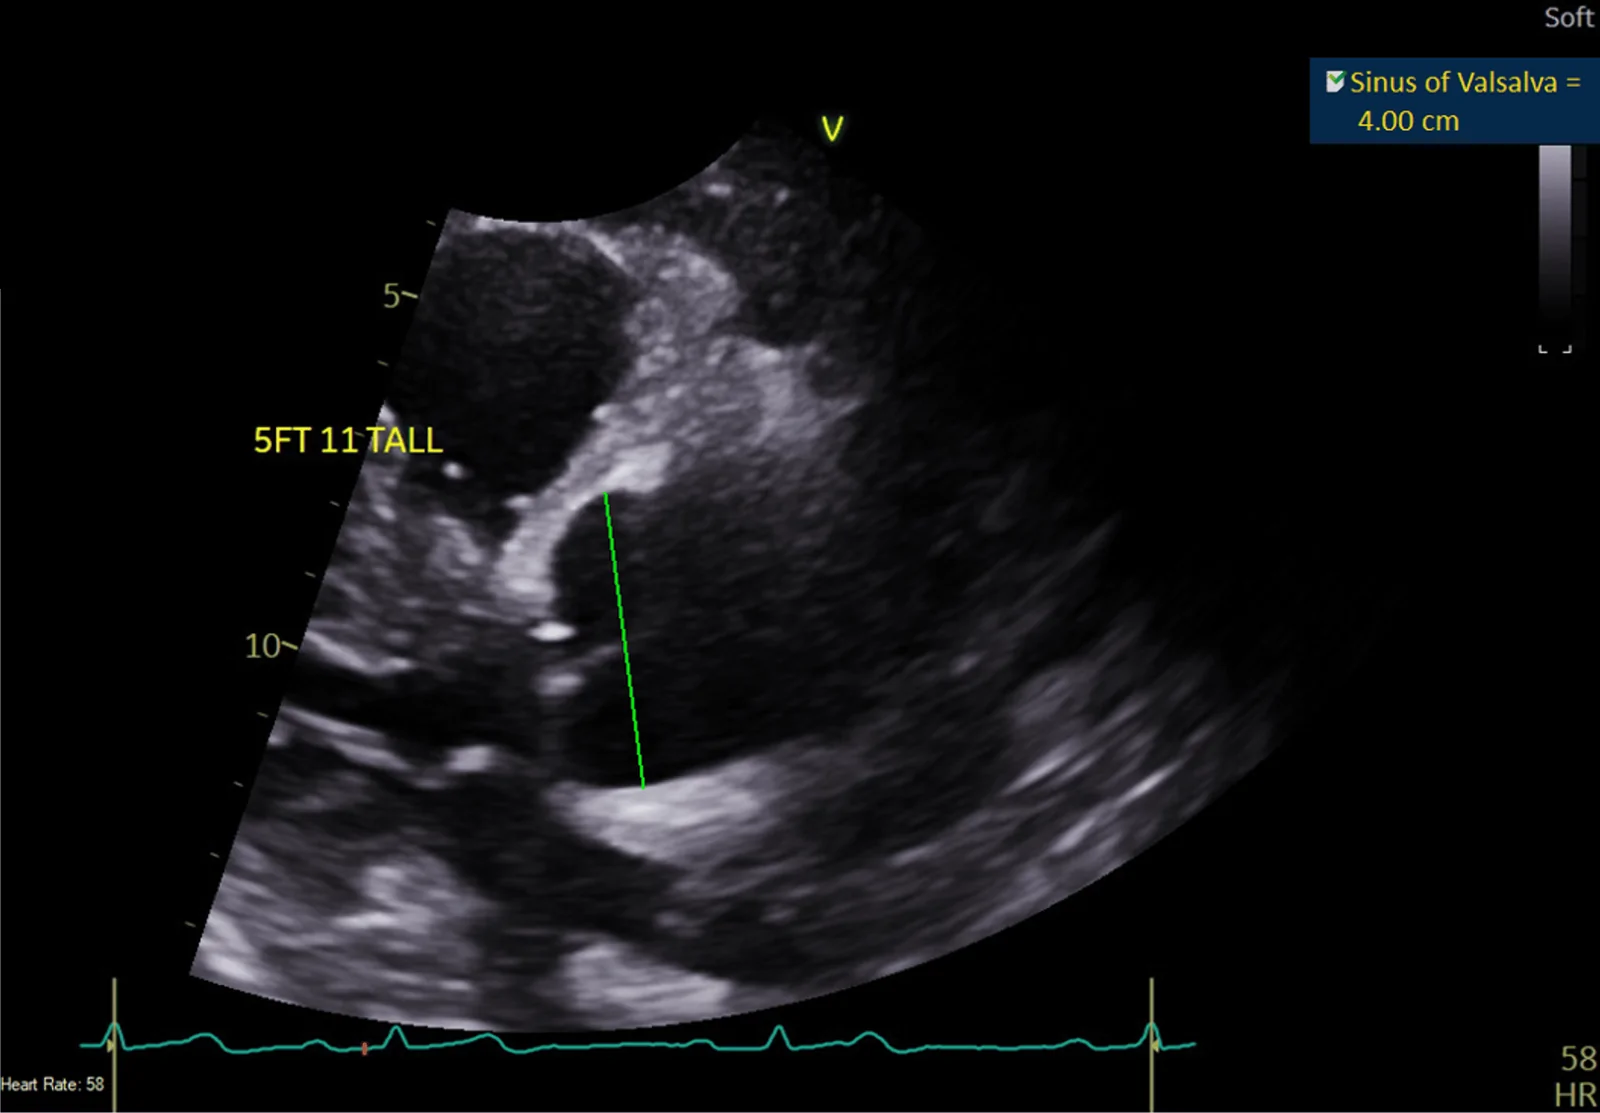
Transthoracic echocardiography (TTE) image showing dilated aortic root.
